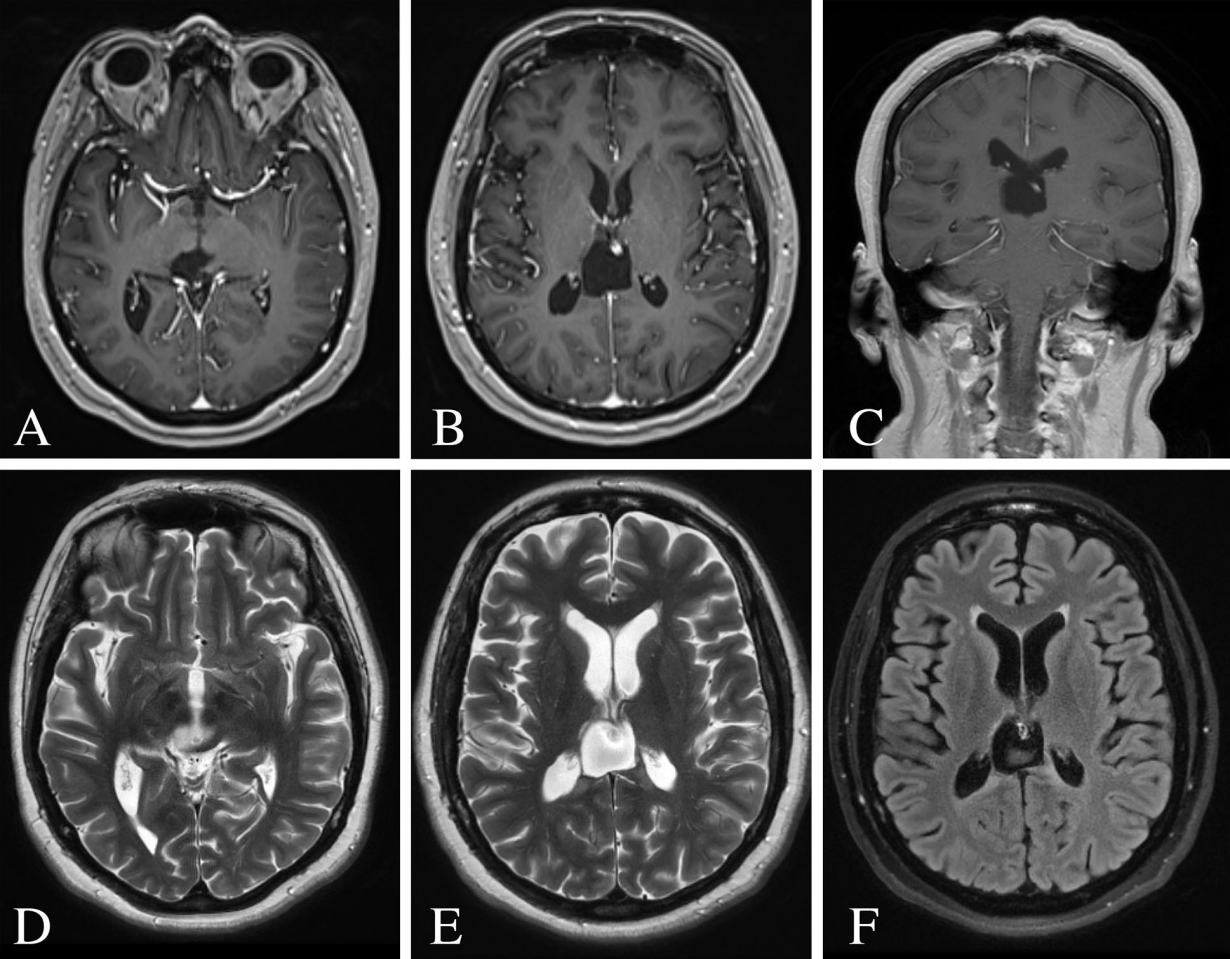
Post-operative MRI of the brain 12 months following surgery. (A–C) Post-contrast T1-weighted images demonstrating no evidence of residual or recurrent disease. (D, E) T2-weighted images showing resolution of the ventricular dilatation and flow voids from the internal cerebral veins. (F) FLAIR image showing minimal hyperintense signal surrounding the surgical resection cavity.
